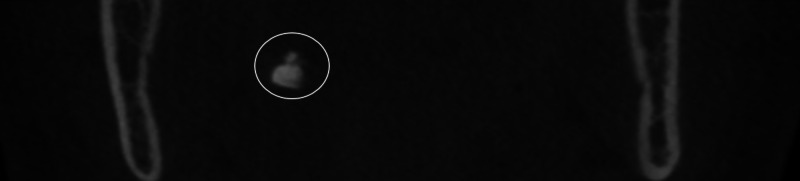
Tonsilloliths in a 60-year-old asymptomatic male A 60-year-old asymptomatic male who came for implant restorative treatment. The frontal cut of the CBCT of the mandible (FOV: 6 cm) shows single and unilateral tonsilloliths calcification (white circle). CBCT: cone-beam computed tomography; FOV: field of view
